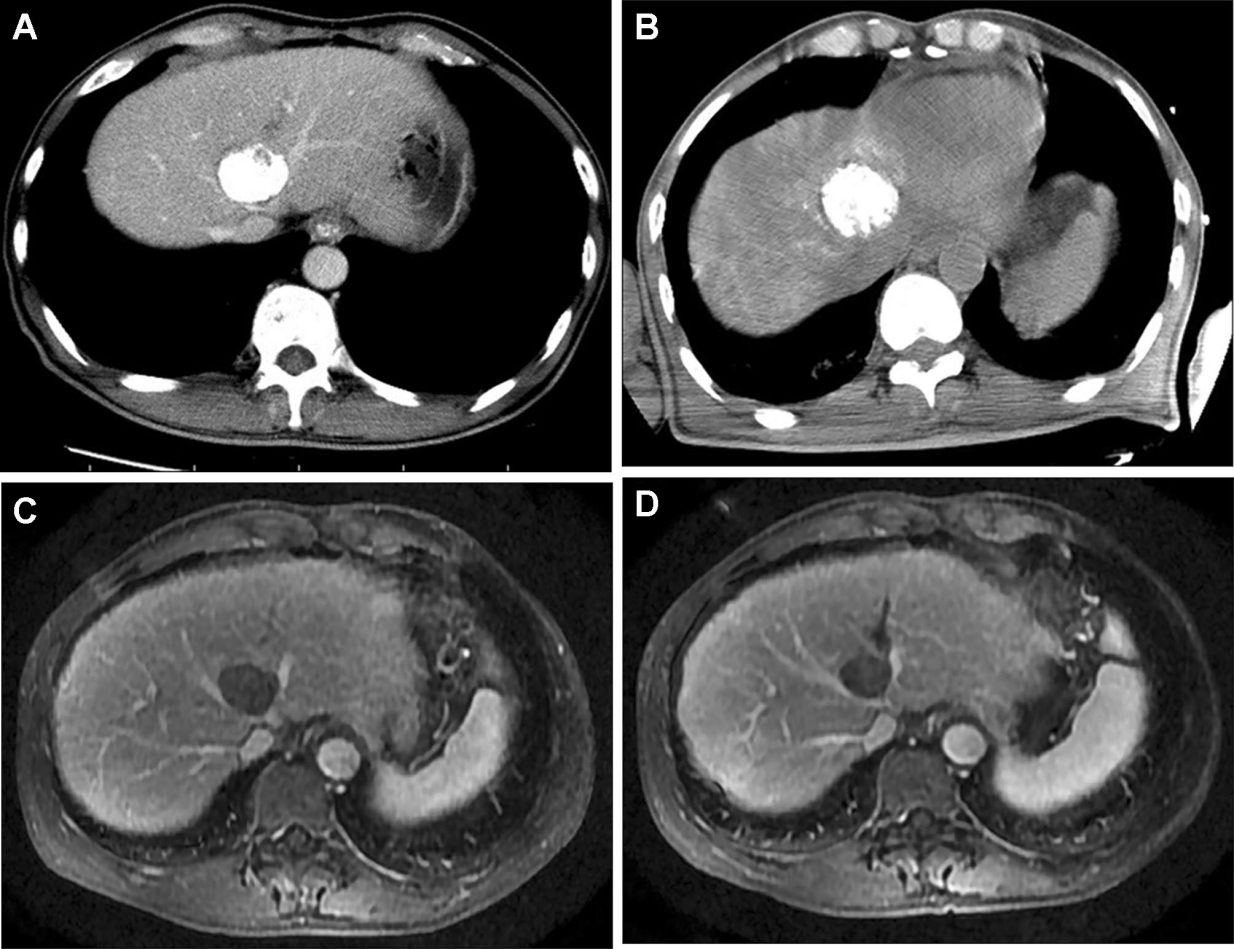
A 60-year-old man with a 47 mm HCC tumor who underwent combination TACE and RFA treatment. A Five days after the TACE procedure, a CT scan was obtained before the RFA procedure. The scan showed a good iodine deposition in the tumor. B During the 1-month follow-up, the contrast-enhanced CT scan showed a decrease in tumor size. C An enhanced MR image 3 months post treatment showed that there was no tumor survival or vascular injury around the tumor. D 27 months post procedure, the MR image showed a maintained decrease in tumor size and no tumor recurrence
